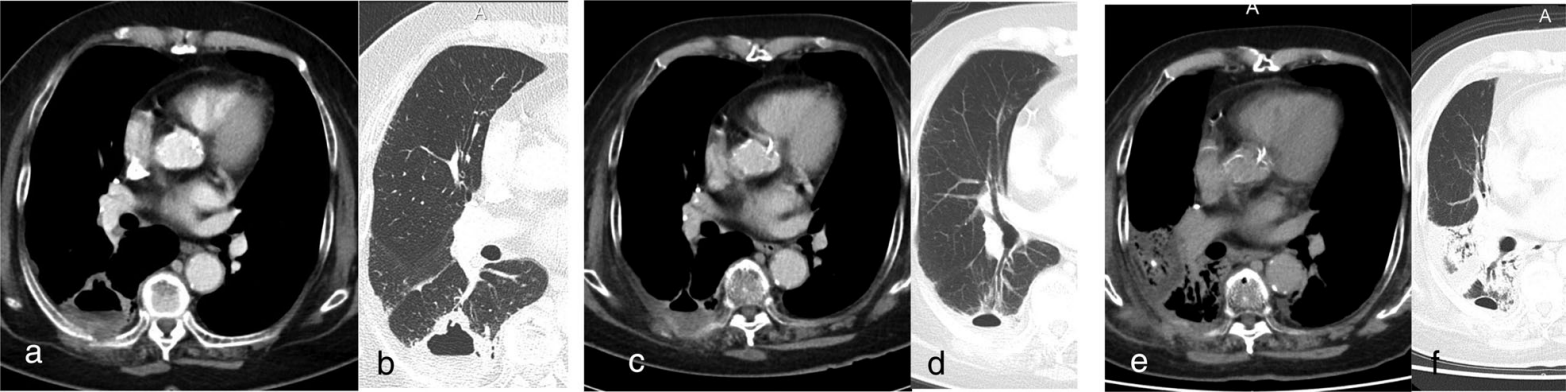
Changes on computed tomography (CT) scan. (a, b) Before radiation therapy. (c, d) Radiation pneumonitis observed after radiation therapy. (e, f) A partial response was evident after four cycles of pembrolizumab. The size of the tumor shrunk from 52 mm to 37 mm after radiation therapy. After four cycles of pembrolizumab the size of the tumor was 28 mm.
